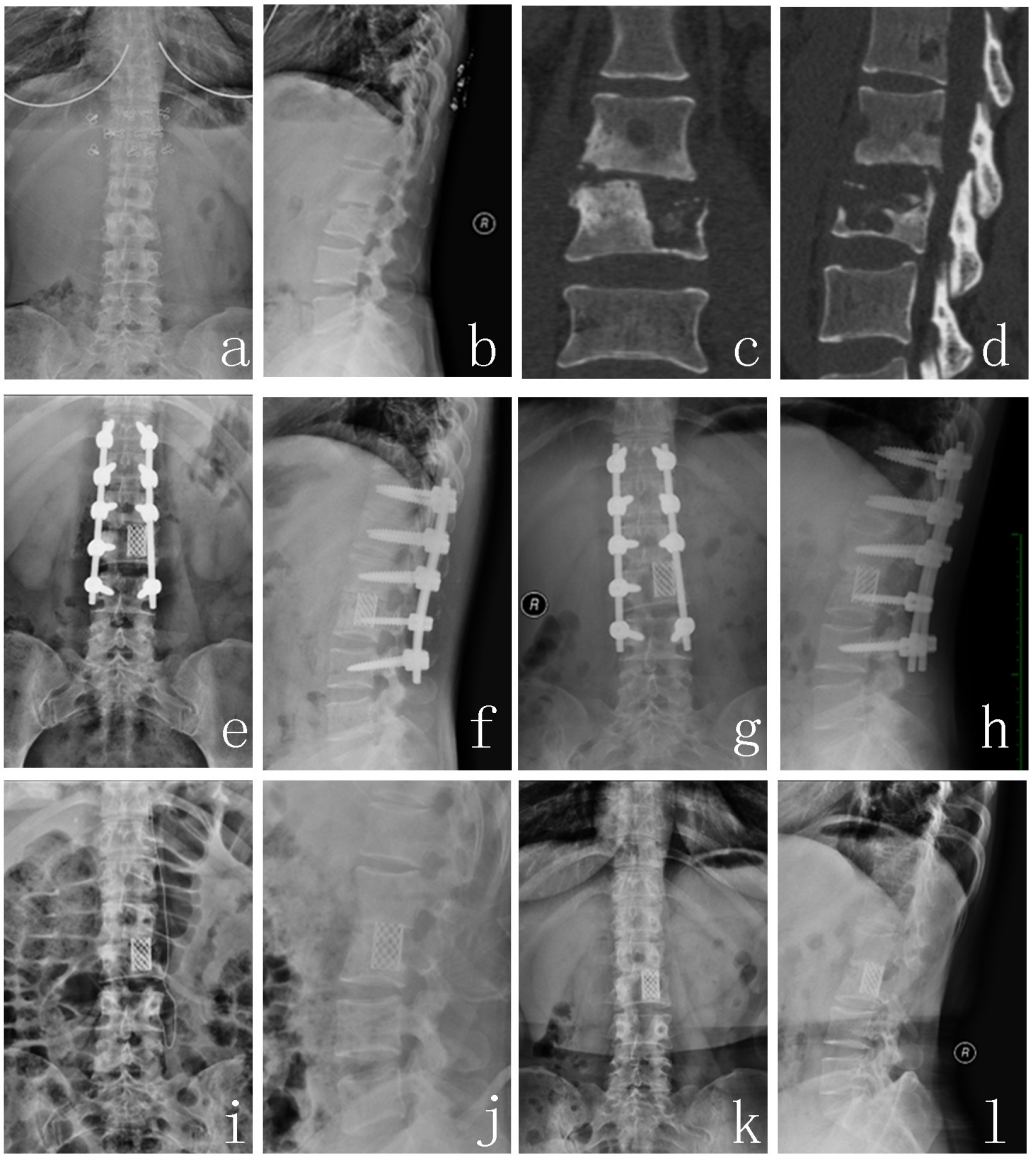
A 36-year-old female patient with L2-3 spinal tuberculosis (Frankel grade E) obtained formed titanium mesh for reconstruction. (a~d) Preoperative X-ray and CT showed that L2 and L3 vertebra body and the intervertebral disc were destroyed, and lumbar instability was formed. (e, f) Postoperative X-ray. (g, h) X-ray at 36 months after surgery. (i, j) Removing the internal fixation at 36 month postoperatively. (k, l) X-ray of 1 year after implant removal showed good location of titanium mesh and lumbar physiological curve.
